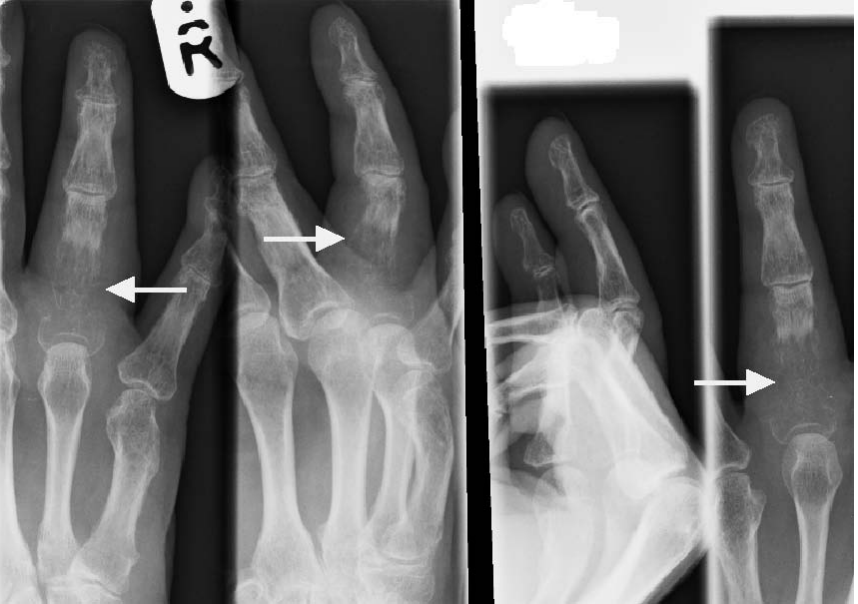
X-Ray of the right hand showing destruction of the proximal phalanx of the ring finger (arrows).
